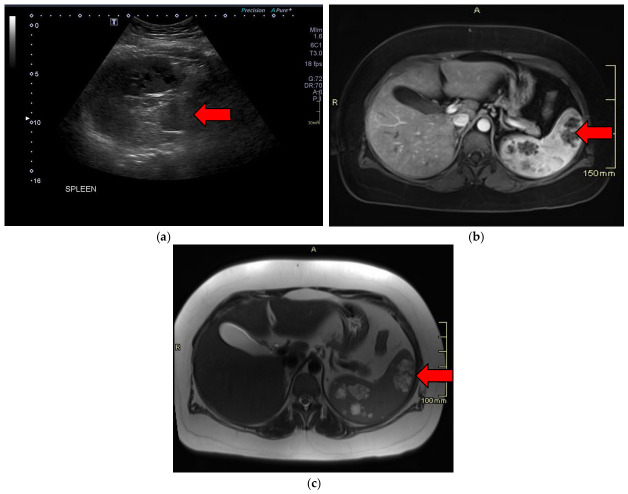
34-year-old female with incidental splenic lesion. (a) On long-axis ultrasound of the spleen, multiple hypo-echoic and anechoic cystic lesions with thin septations. (b) On an axial T1, the spleen contains well-circumscribed multiloculated cystic lesions that are hypo-intense. (c) On an axial T2, the lymphangioma demonstrates multiple well-defined “honeycomb” like masses with high signal intensity and are thin-walled.
